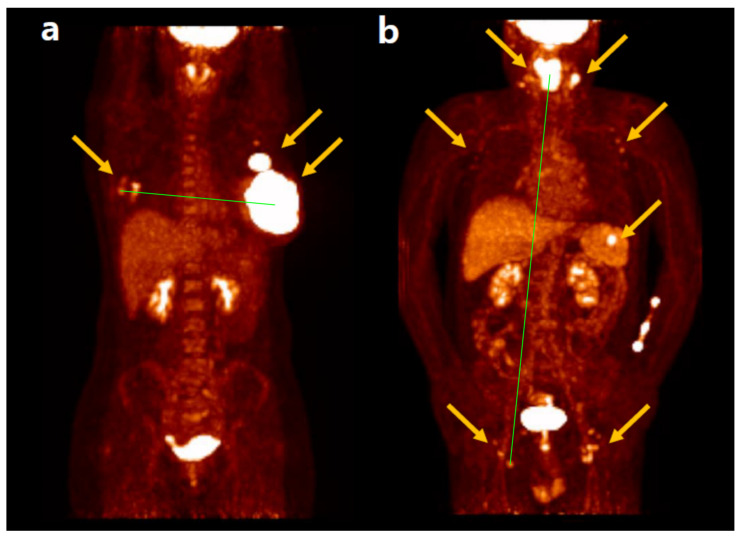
FDG PET/CT images of patients with DLBCL (indicated by arrows) who have known high-risk factors for relapse, in addition to Dmax (line). (a) A 38-year-old woman with Ann Arbor stage IV, ECOG PS 0, serum LDH 969 IU/L, IPI score 3, SUVmax 35.4, TMTV 362 mL, tTLG 8287, interim DS 4, EOT DS 4, and Dmax 253.1 mm. No recurrence was found until the last clinical follow-up at 107.7 months. (b) A 61-year-old man with Ann Arbor stage III, ECOG PS 1, serum LDH 461 IU/L, IPI score 3, SUVmax 11.4, TMTV 71.8 mL, tTLG 352.5, interim DS 2, EOT DS 1, and Dmax 743.9 mm. Recurrence was found after 16.6 months of follow-up. He survived until the last clinical follow-up at 77.3 months.
